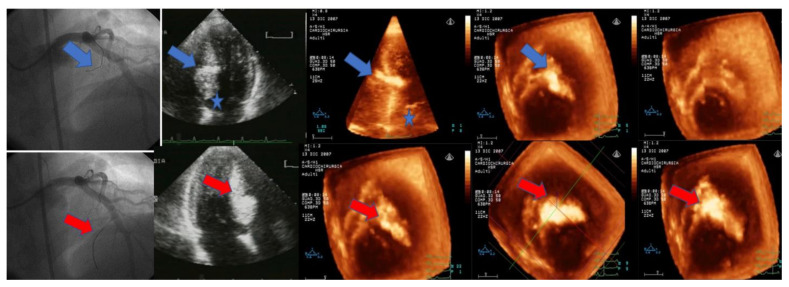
Echocardiographic images of intracoronary MCE in a patient with HCM. Following echo-contrast agent injection into the first septal coronary branch, MC-DE shows a contrast effect in the mid-interventricular septum outside TSZ, confirmed by subsequent MC-3E analysis with multiple slice reconstruction from base to cardiac apex (Type 3 pattern); following subsequent echo-contrast agent injection into the second septal coronary branch, MC-2DE images reveals a large septal opacification, which is confirmed by MC-3DE multiple reconstruction showing myocardial contrast effect extension along the overall interventricular septum (*Type 3 pattern*). Owing to the high risk of extended septal myocardial infarction, the patient was considered unsuitable for ASA.
